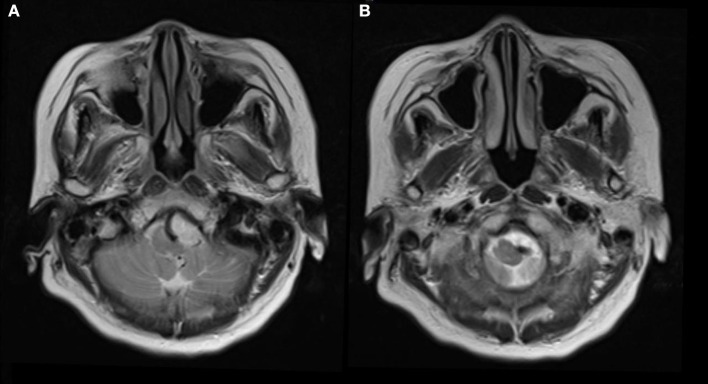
Seventy-three-year-old woman presented with vertigo and imbalance. Magnetic resonance imaging showed severe compression and indentation (A) of the left lower medulla. Note that the medulla was displaced to the right side (B) by the tortuous vertebral artery.
